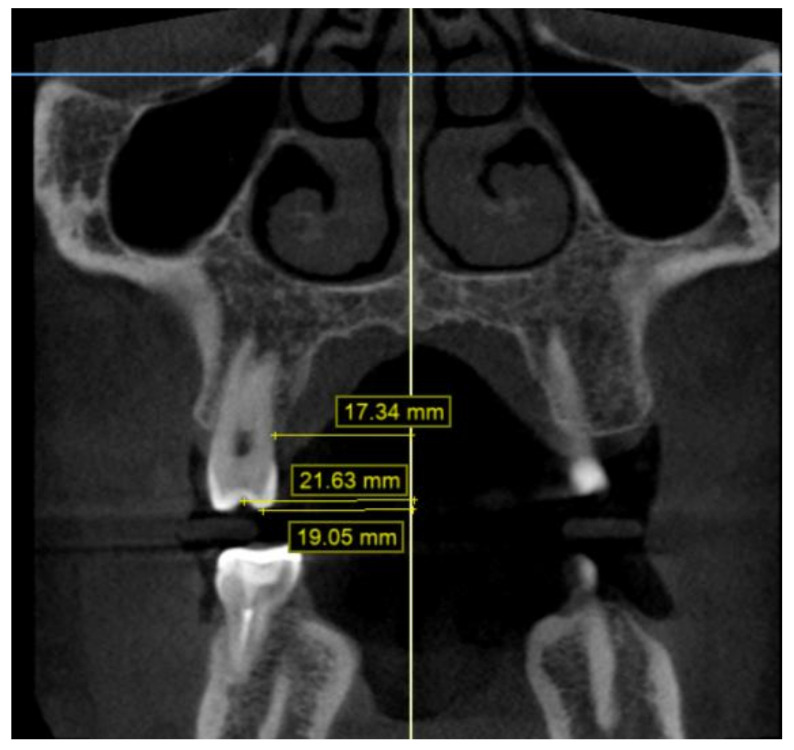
Distances from second premolar to medial sagittal plane on CBCT scan: groove, lingual gingival, and palatal cuspid.
